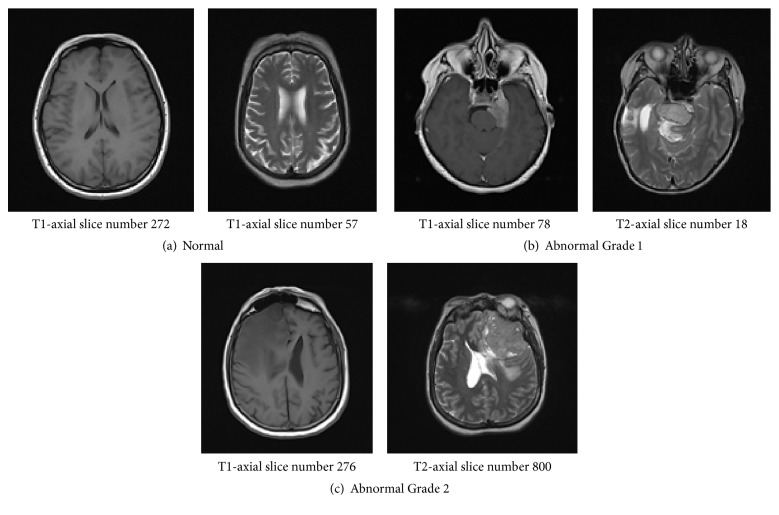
Examples of axial sequences in (a) normal and ((b) and (c)) abnormal brain MR images (i) T1-axial and (ii) T2-axial. Source: collected data from UKM Medical Center.
